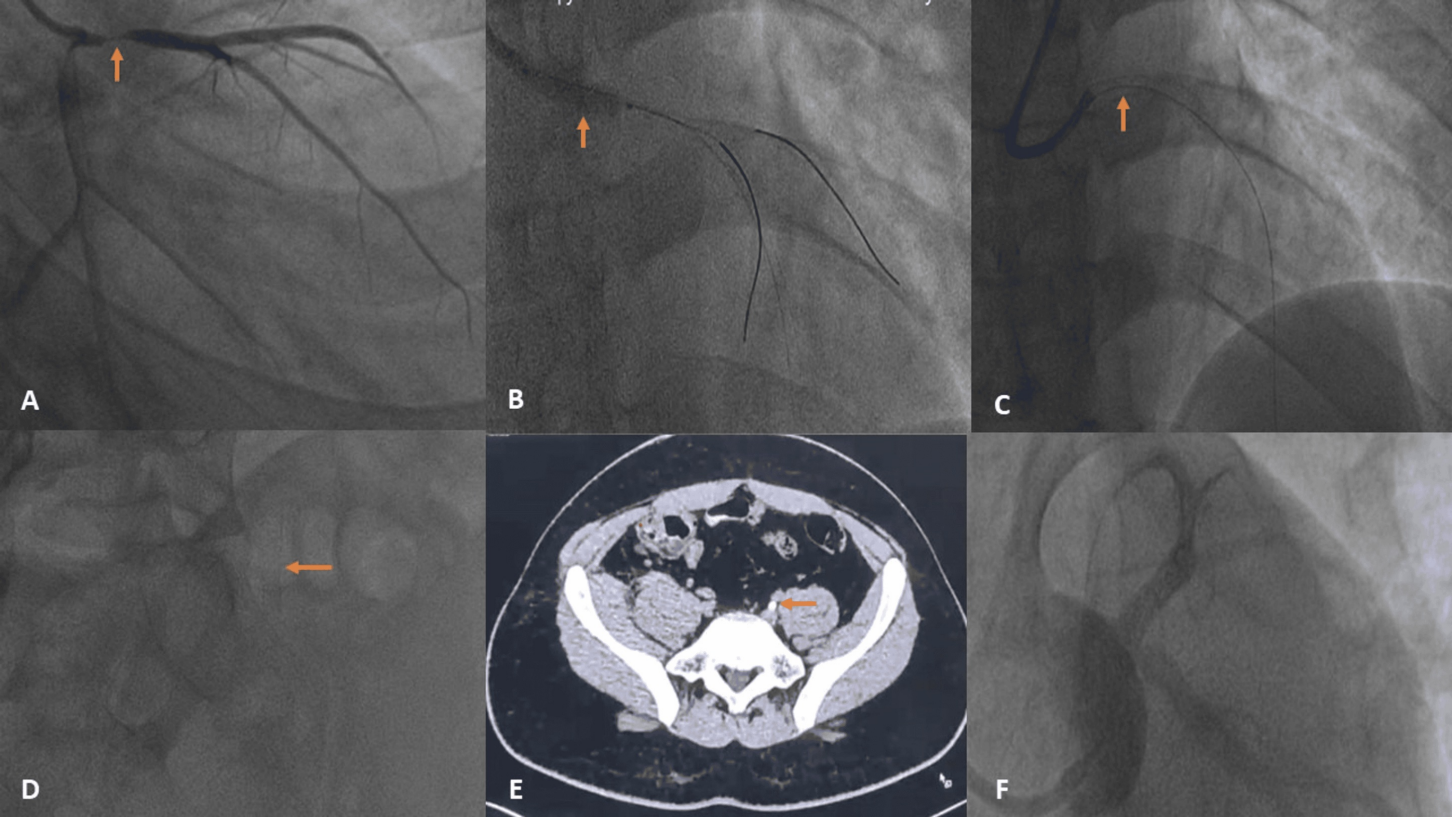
Coronary angiograms, fluoroscopy, and NCCT images. (A) Coronary angiogram of the left coronary system taken in the right anterior oblique (RAO) caudal view, which reveals a tubular lesion (arrow) in the ostio-proximal left anterior descending (LAD) artery extending into the distal left main coronary artery. (B) Coronary angiogram in the left anterior oblique cranial view with stent being deployed from left main ostia to the ostio-proximal LAD (arrow). (C) The result post-deployment of the stent in left anterior oblique (LAO) cranial view (arrow). (D) The left main stent has embolized to the left common iliac artery as seen on fluoroscopy (arrow). (E) The stent (arrow) in a cross-sectional view on NCCT of the abdomen and pelvis. Part F reveals the final result of the procedure after deploying the second stent in the left main coronary artery in the LAO caudal view with TIMI 3 flow. NCCT: non-contrast computed tomography; TIMI: thrombolysis in myocardial infarction
